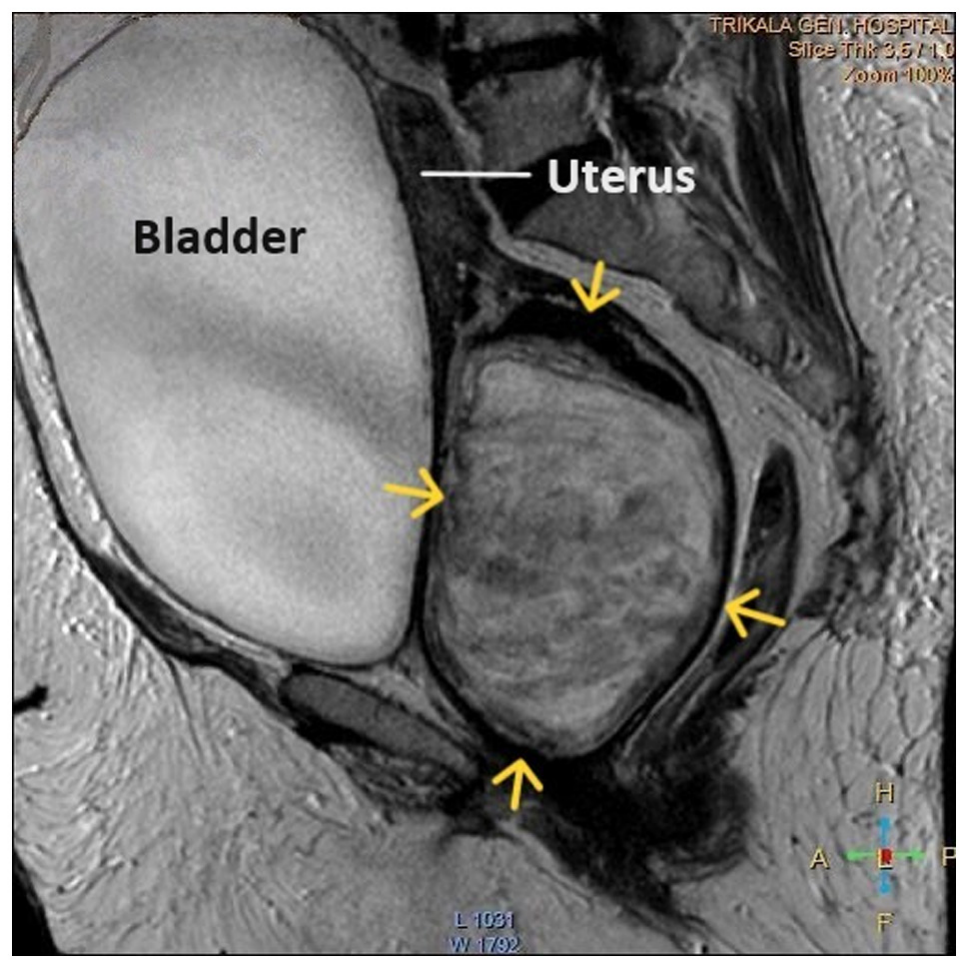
Magnetic resonance imaging of a large prolapse pedunculated submucosal uterine leiomyoma: The depicted urinary bladder distention with internal non-homogeneity of the cervical canal and presence of a lobulated lesion in the endocervix (yellow arrows) was misdiagnosed as an intracervical leiomyoma.
